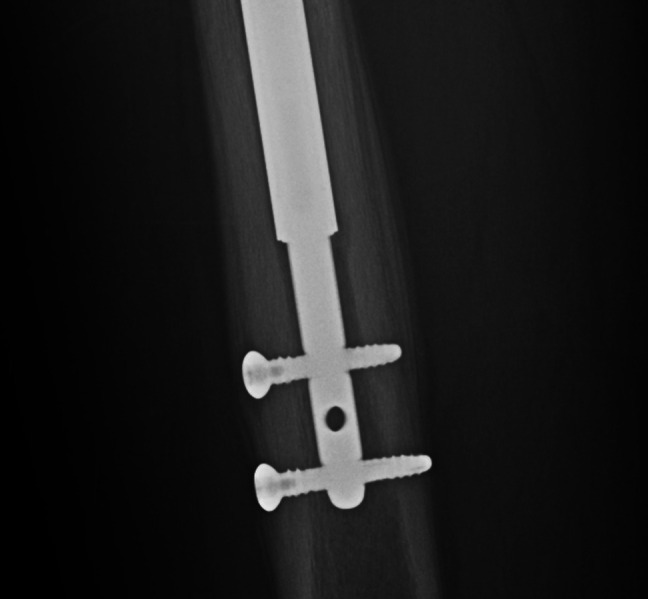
AP radiograph (with 135% magnification) of a right femur demonstrating that a punched-out appearance resembling osteolysis is appreciated medial to the modular junction. Onion skinning resembling periosteal reaction is appreciated along the medial femoral shaft adjacent to the modular junction and around both ends of the distal locking screws.
